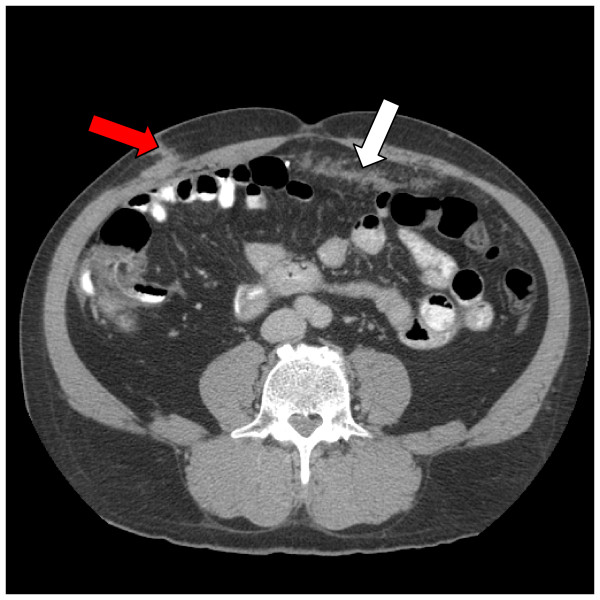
Computed tomography (CT) scan of the abdomen. This CT scan of the abdomen performed before cytoreductive surgery (CRS) and hyperthermic intraperitoneal chemotherapy (HIPEC) shows neoplastic involvement of trocar sites (red arrow) and omentum (white arrow).
